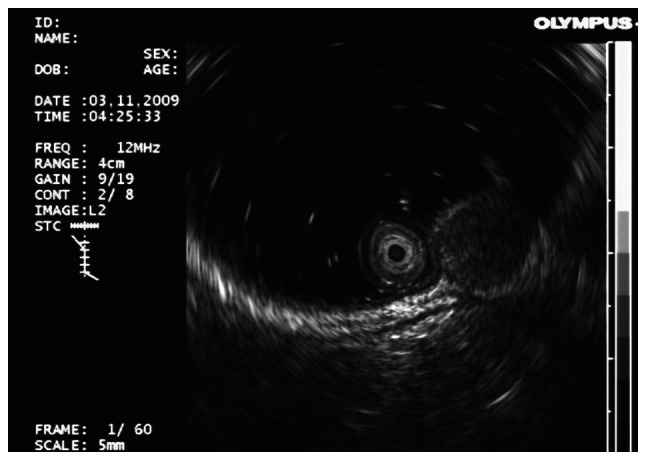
Appearance of rectal carcinoid under endoscopic ultrasound demonstrates hypoechoic nodules located in the submucosa with clear boundaries.
